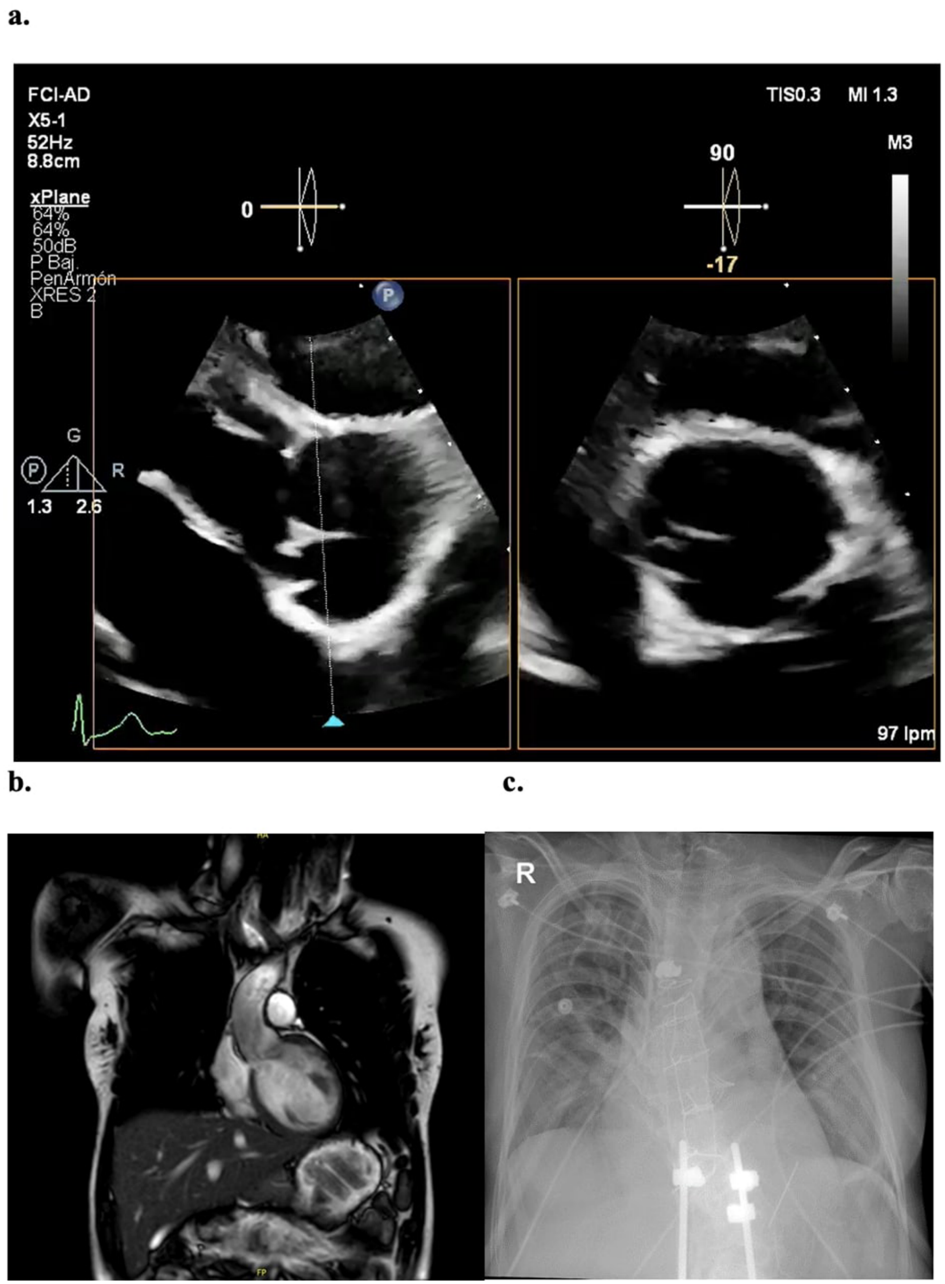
Imaging studies (a) preoperative transthoracic echocardiogram, with severe aortic regurgitation associated to a non-coronary cusp retraction. (b) Coronal view of Cardiac MRI, showing a non-dilated ascending aorta with maximum commissural diameter of 30 mm (c) postoperative x-ray evidencing 6 surgical steel wire sutures and sternal hemi cerclage.
